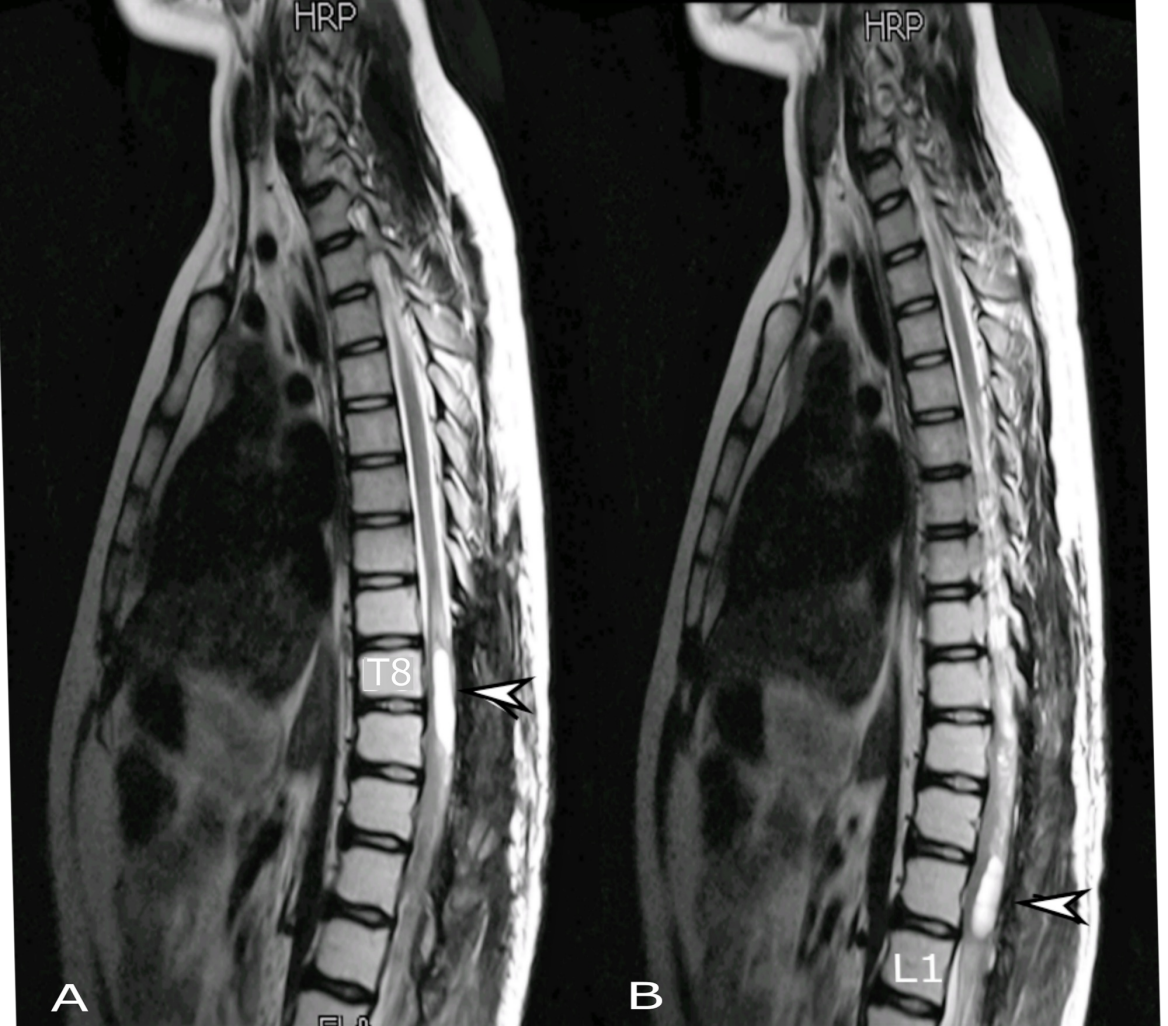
Post-radiation MRI thoracic spine, sagittal section. T2 weighted image showing development of cystic degeneration at (A) T8-T9 and (B) T12-L1 (arrows). MRI: Magnetic resonance imaging.
